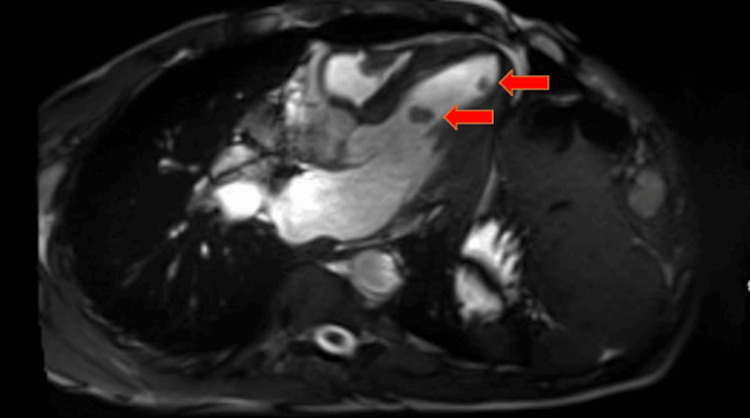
Cardiac MRI revealing two new hypodense filling defects within the left ventricular chamber concerning for intracardiac metastasis
